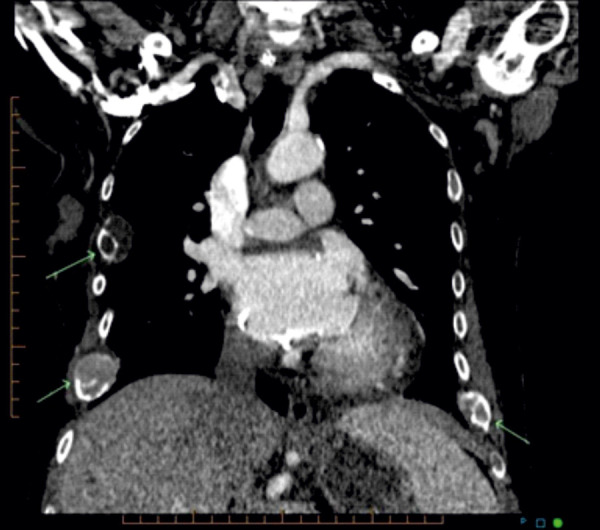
CT of the chest: Lytic bone lesions in ribs with soft tissue masses.
